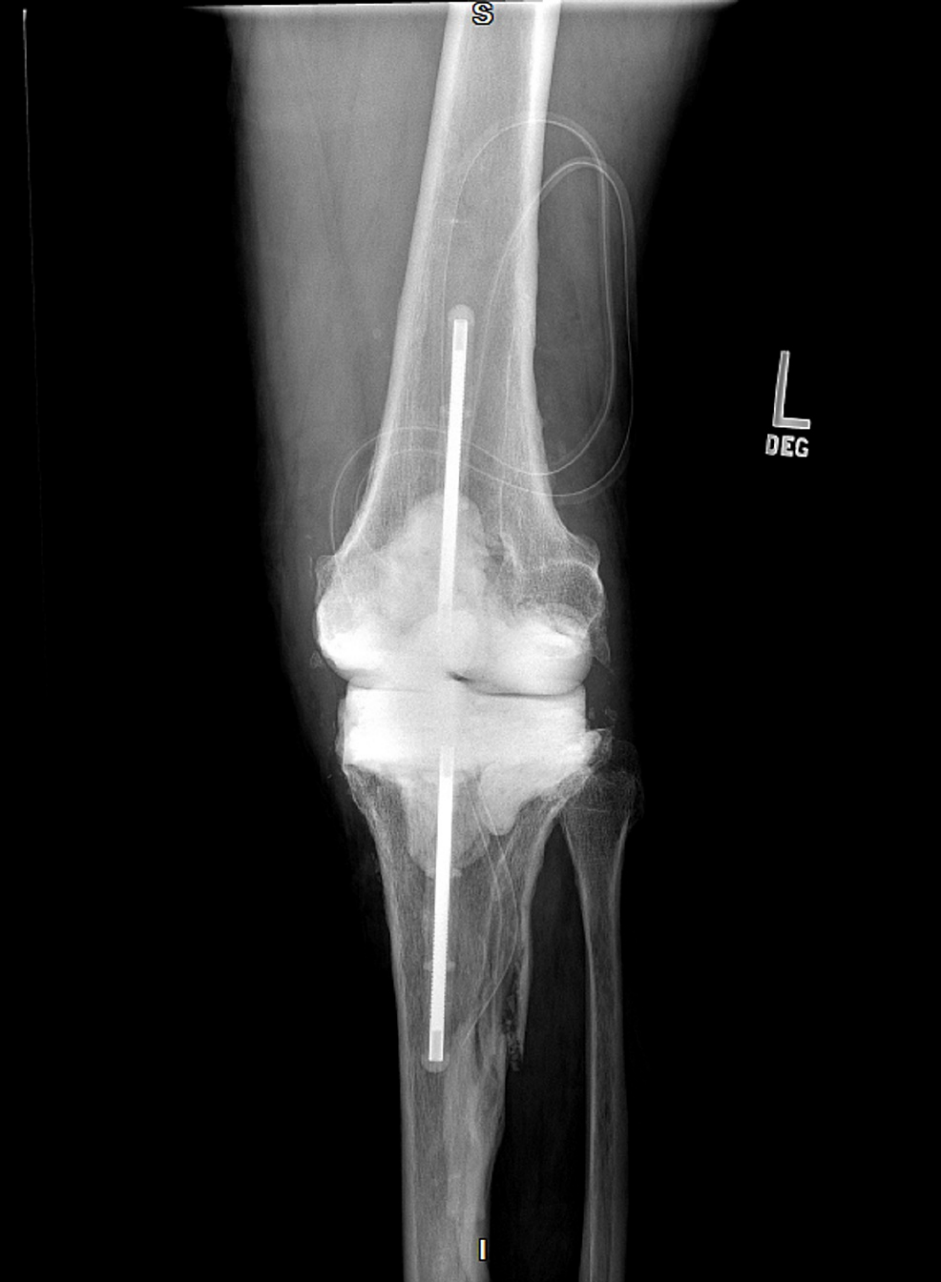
X-ray of the infected left knee post-surgical antibiotic knee spacer (anteroposterior)
